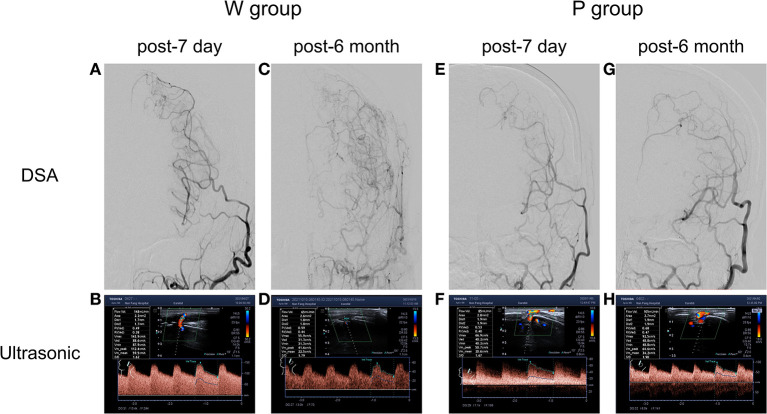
Typical STA grafts with different groups showed at post-operate 7 day and post-operate 6 month. In well-compensated collateral group (W group), (A) post-operative 7 day DSA showed the patency of grafts, (B) with a high flow rate of 148 ml/min in the ultrasonography examination. (C) Post-operative 6 month DSA confirmed a well-compensated collateral, (D) with a flow rate of 65 ml/min in the ultrasonography examination. In poorly-compensated collateral group (P group), (E) post-operative 7 day DSA showed the patency of grafts, (F) with a high flow rate of 85 ml/min in the ultrasonography examination. (G) Post-operative DSA confirmed a poorly-compensated collateral, (H) with a flow rate of 105 ml/min in the ultrasonography examination.
